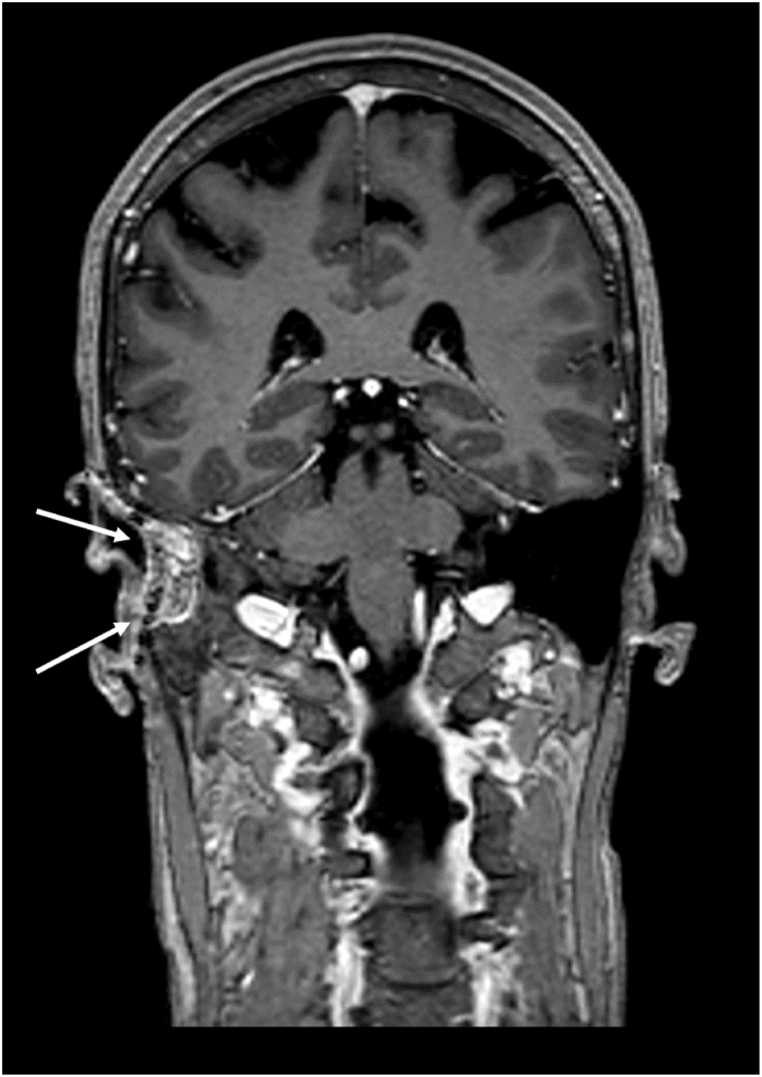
Coronal reconstruction of T1-weighted imaging after gadolinium. The residual meningioma is not visible. Hyperintense (fatty) tissue after subtotal petrosectomy on the right side (white arrows). On the left side normal aspect of air containing temporal bone and middle ear.
